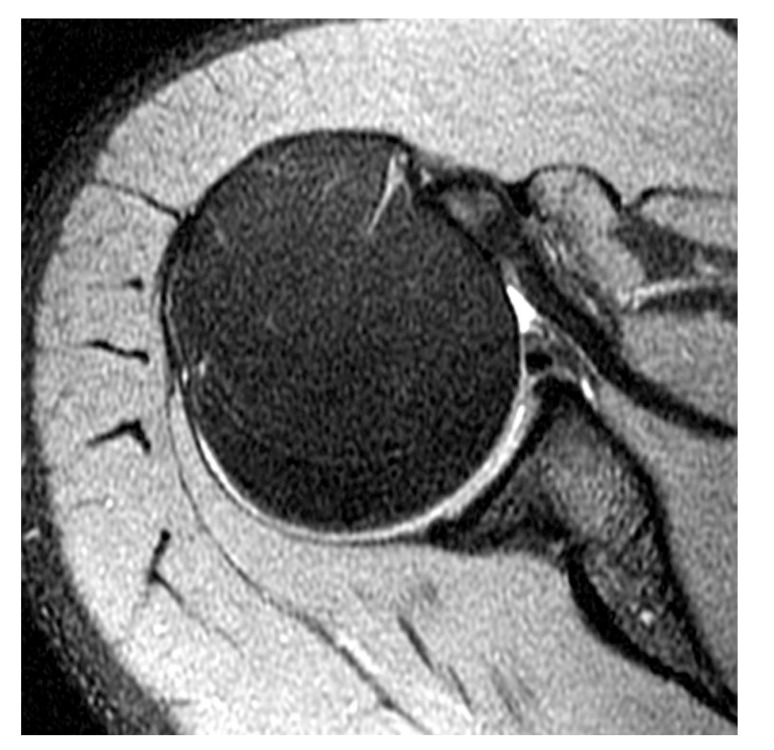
Sublabral foramen. (A) Axial fat-suppressed T2-weighted image of a 29 year-old male athlete with smoothly marginated, full-thickness separation of the labrum isolated to the anterosuperior quadrant.
